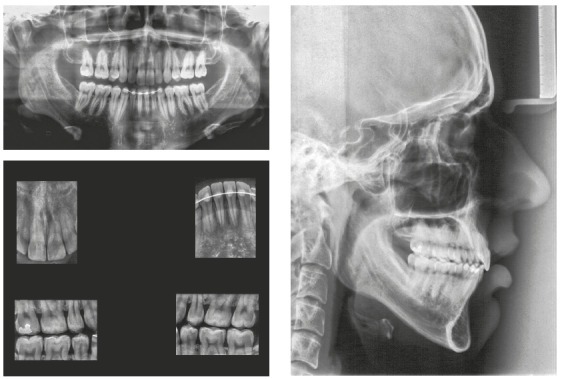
Posttreatment radiographs at 2 years follow-up.
